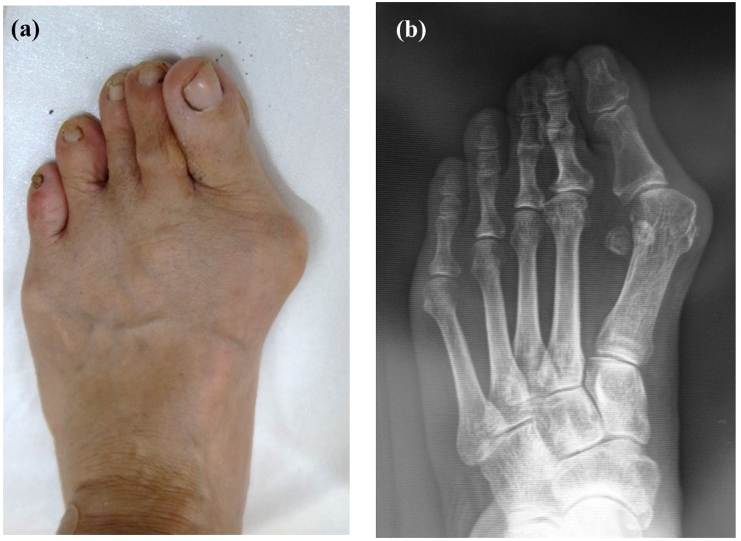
Preoperative photograph (a) and radiograph (b) of the foot in a patient with hallux valgus.
